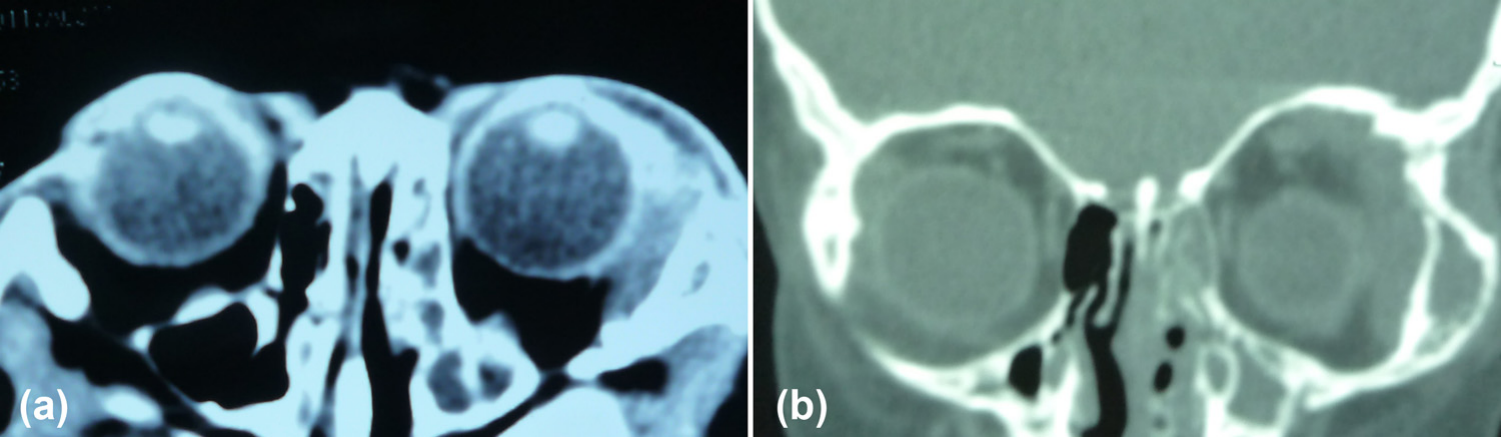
The radiological findings of the 2-year-old girl with an orbital mass. (a) Orbital axial CT showing the soft tissue mass on the lateral orbital rim of the left eye; (b) orbital coronal CT in the bone window showing the soft tissue mass on the lateral superior orbital rim of the left eye, with bone destruction in the lateral wall of the left orbit.
